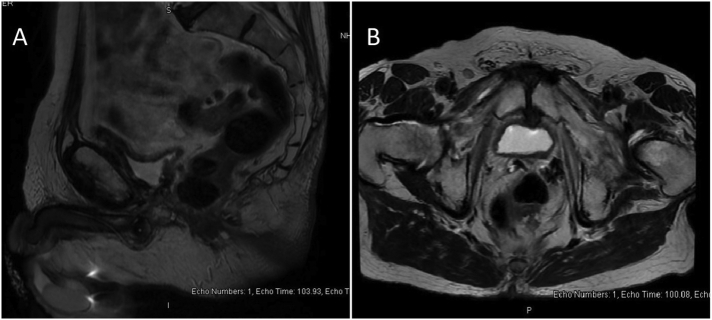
Pelvic magnetic resonance imaging (A) sagittal, (B) axial T2-weightted views showing abnormal posterior wall thickening within the lower rectum, around 2 cm above the anal canal and irregular outlines within the mesorectal soft tissue.
